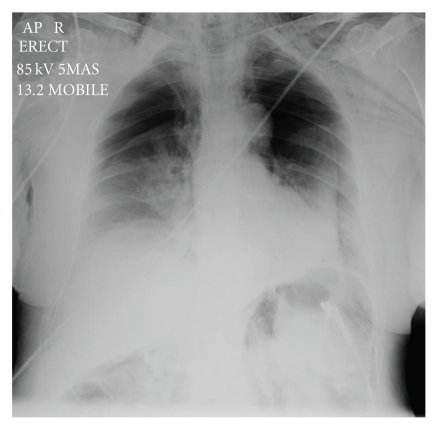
Chest X-Ray prior to chest drain insertion. Note the bilateral pneumothoraces.
